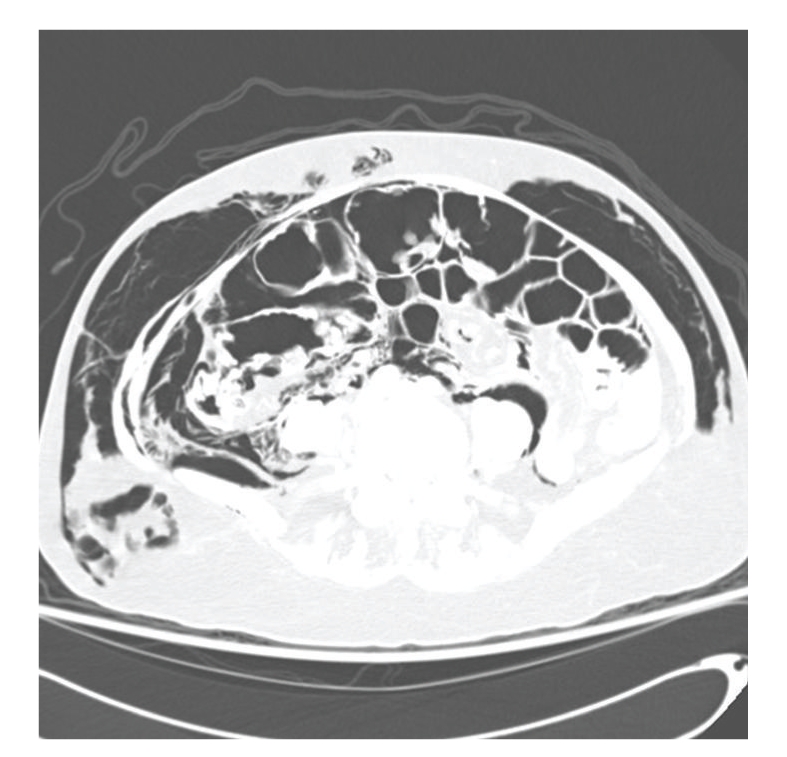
Transverse section of CT abdomen showing subcutaneous emphysema and pneumoperitoneum.
